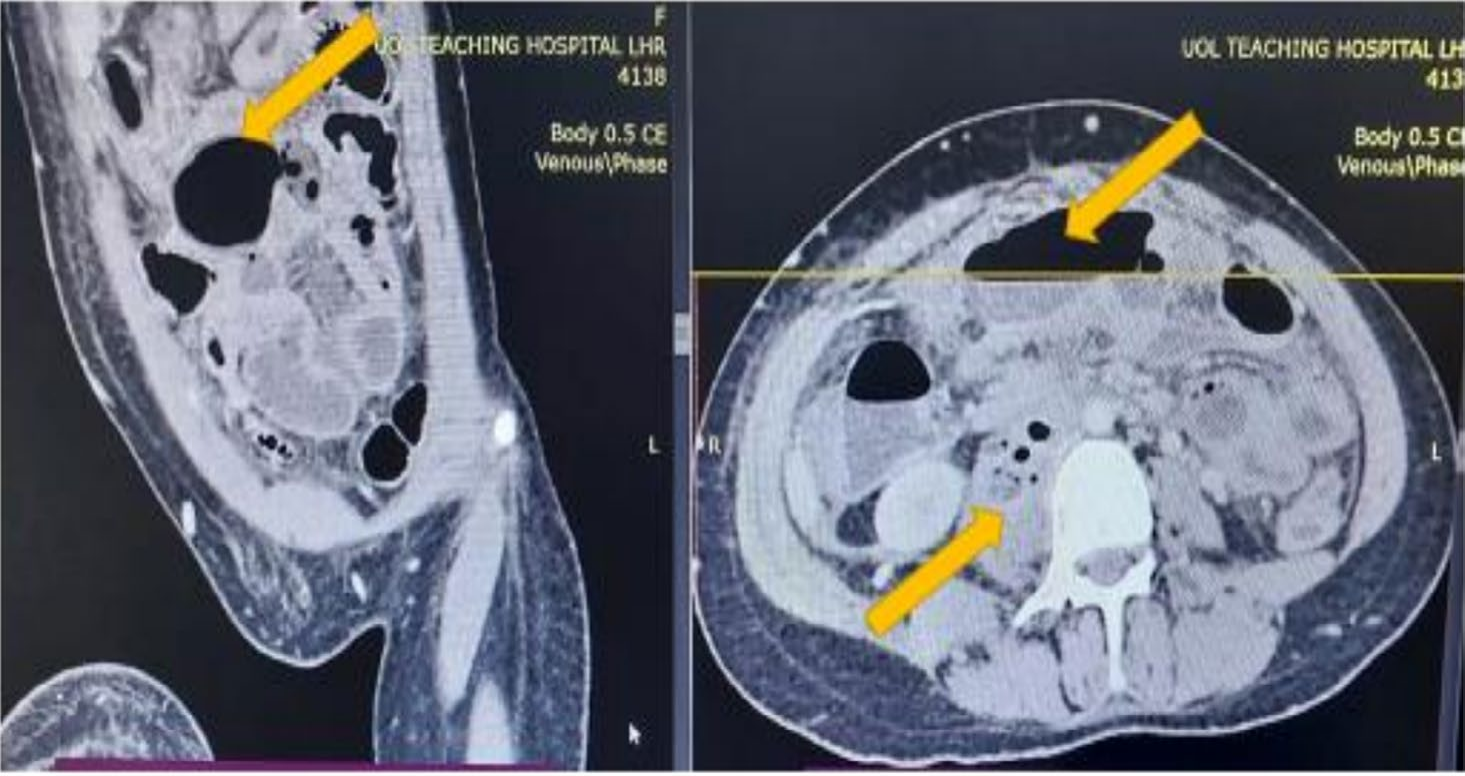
Pathognomonic finding of air in the inferior vena cava. A coronal reformatted contrast‐enhanced CT image clearly showing multiple locules of intraluminal gas within the inferior vena cava (IVC). This finding is pathognomonic for an enterovascular fistula. The large, rim‐enhancing right psoas abscess is also visible.
